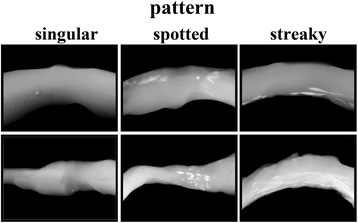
Exemplary samples of digital contact radiography images of the acetabular labrum from six different donors with distinct cartilage calcification. Cartilage calcification was detected as radiopaque spots within the fibrocartilage of the acetabular labrum. The cartilage calcification was classified into three typical calcification patterns (singular, spotted and streaky)
